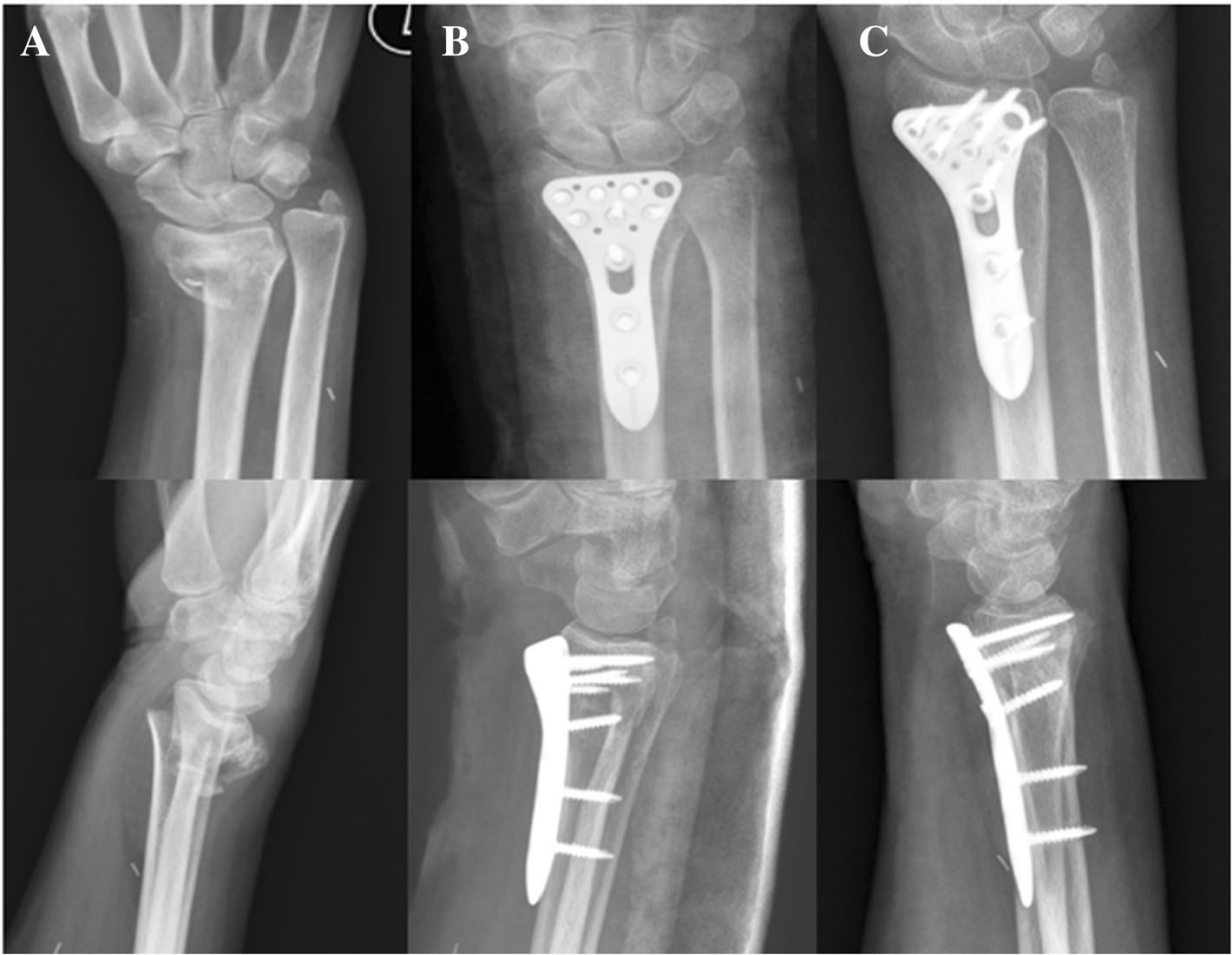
The 67-year-old female with left distal radius fracture (AO type C2) and the ipsilateral brachiocephalic shunt was treated with a volar locking plate. The serial images are preoperative (a), immediately postoperative (b), and 1-year follow-up (c) radiographs. The upper row was the anteroposterior view and the lower row was the lateral view
